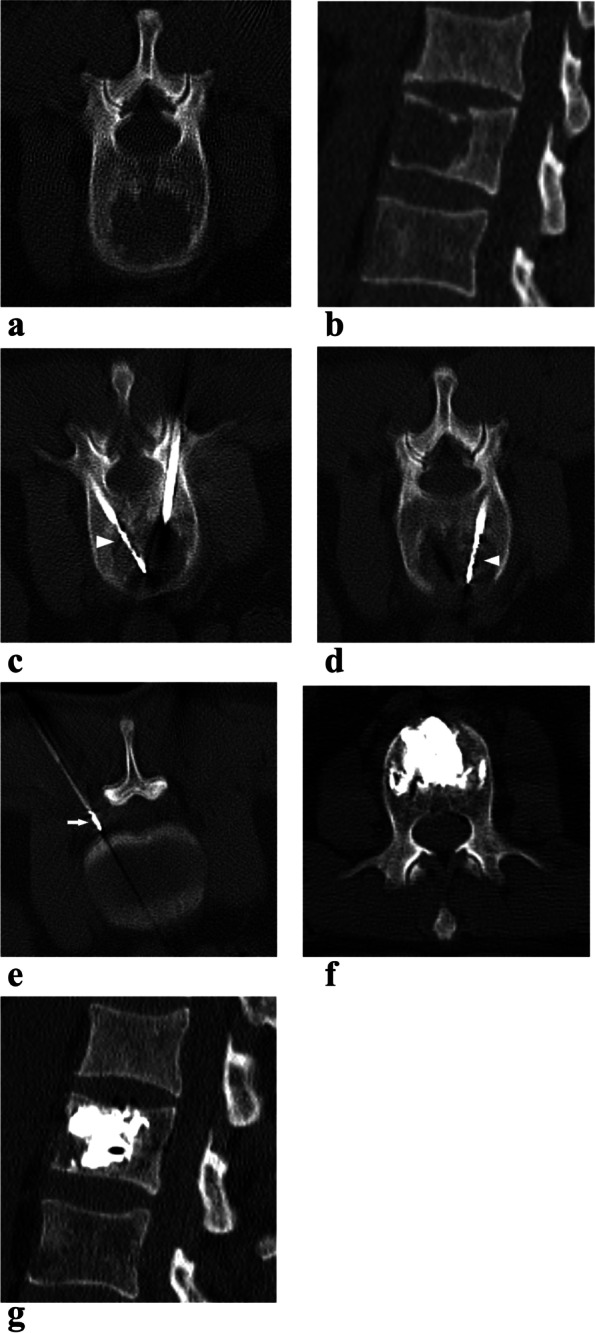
A 58-year-old man with lung adenocarcinoma with L2 osteolytic metastasis (recurrence after radiotherapy) treated with MWA in combination with cementoplasty. L2 osteolytic metastasis showed on axial and sagittal reconstructed CT (a, b). The microwave ablation antennas (arrowheads) were inserted into the lesion through bilateral access (c, d). The thermometric electrode (arrow) was inserted into the left intervertebral foramen (e). The cement deposited in the vertebral body without significant leakage on axial and sagittal CT (f, g)
